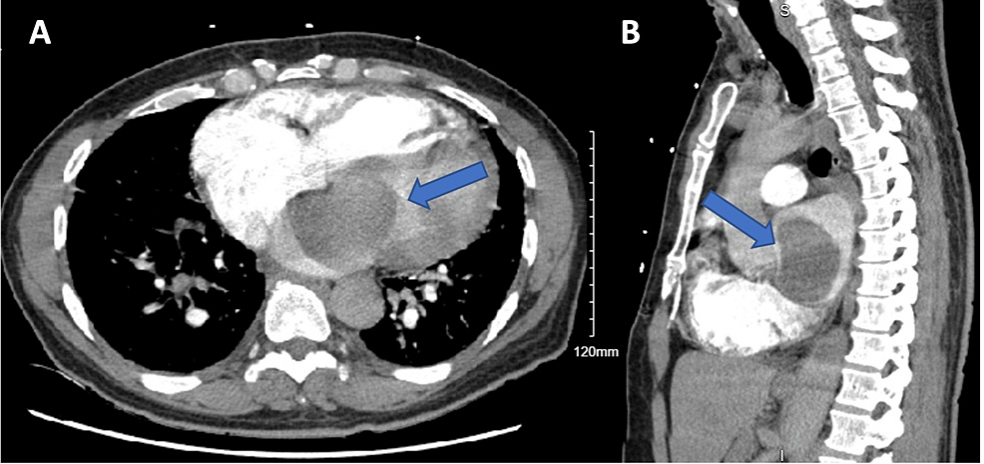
Computed tomography angiography of the chest. Image A shows an axial cross-section of the thorax at the level of the left atrium with a large filling defect reflecting the left atrial mass (arrow). Image B shows a sagittal cross-section with a left atrial filling defect representing mass (arrow).
